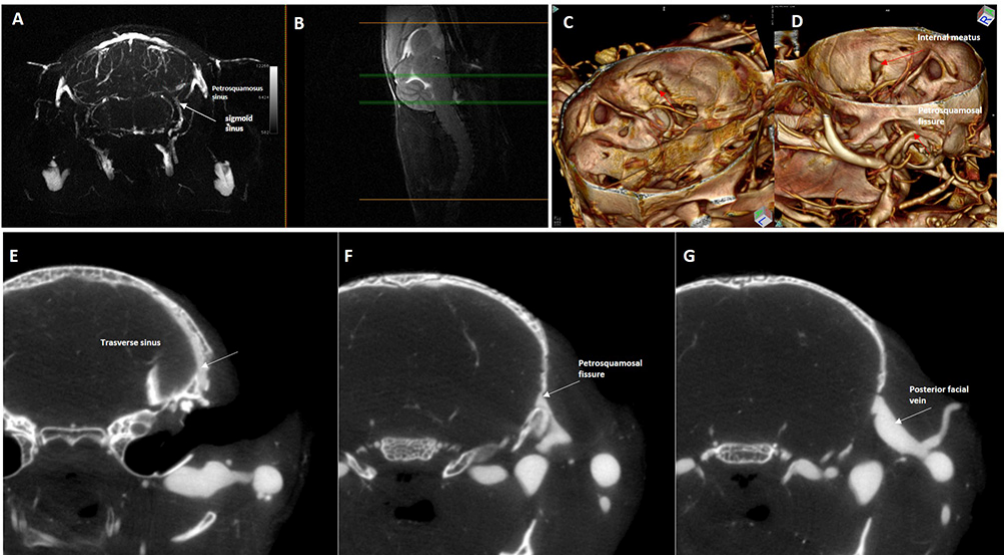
Magnetic resonance angiography with 3D rendering and silicon-computed tomography angiography of the petrosquamosal fissure. Upper row: Maximum Intensity Projection of axial magnetic resonance angiography partitions (slab at the occipito-cerebellar border (B) mid-sagittal T2w slice used as a reference,) and 3D rendering of silicon-enhanced computed tomography angiography as seen from (C) inside and (D) outside of the skull. (E), (F), (G) Lower row: Axial computed tomography slices in caudo-cranial sequence showing the exit from the brain of most of the transverse sinus blood through the petrosquamosal fissure (arrows), then joining the external jugular system.
